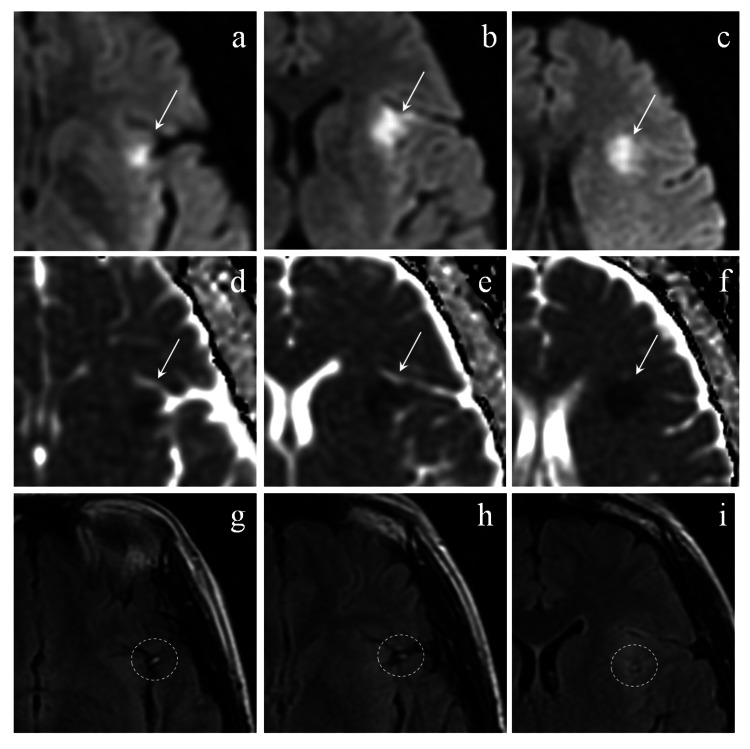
Initial MRI imaging with 6 mm-thickness slices three days after amnestic episode (a-c) Axial diffusion-weighted imaging with the parameters of repetition time (TR)/echo time (TE) = 5,000/84.3 ms. White arrows show hyperintensities in the left anterior insular cortex. (d-f) Axial apparent diffusion coefficient map with the parameters of TR/TE = 5,000/84.3 ms. White arrows show signal loss at the same site of diffusion restriction area. (g-i) Axial fluid-attenuated inversion recovery with TR/TE = 10,000/139 ms. White circles show hyperintense MCA signs in the left Sylvian fissure. MCA: middle cerebral artery; MRI: magnetic resonance imaging
